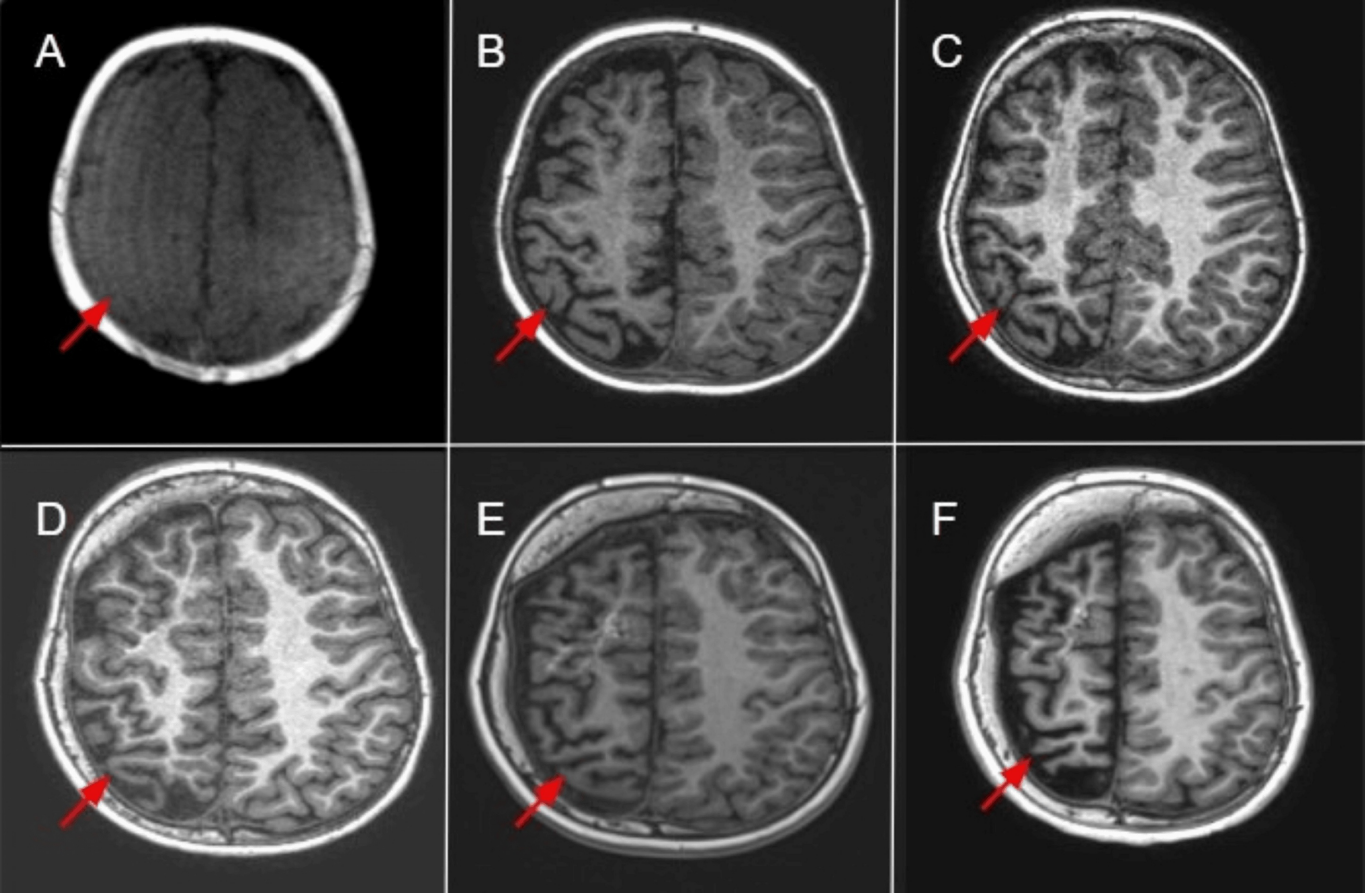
Axial non-fat-saturated T1-weighted images Axial non-fat-saturated T1-weighted images (A–F) demonstrate progressive right cerebral atrophy (red arrows) at one month, one year, five years, six years, 10 years, and 12 years of age, respectively.
